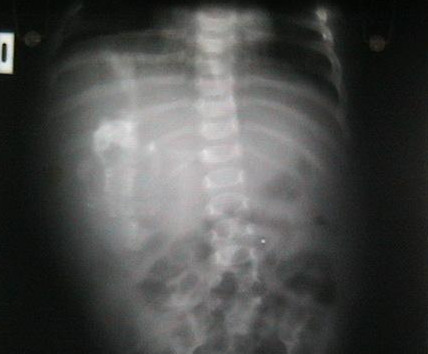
Anteroposterior abdominal radiograph demonstrates a soft tissue mass in the right hemiabdomen. The mass contains calcified osseous-appearing structures of varying sizes and shapes (see arrows)
